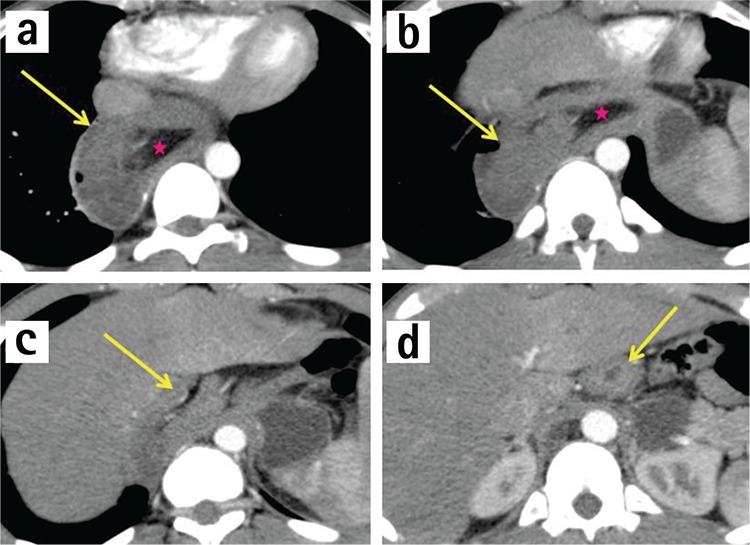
a-d. Axial view, (yellow arrows show stomach layers, red stars omental fat). Contrast-enhanced computed tomography (arterial phase) showed all layers of the stomach and omental fat herniated into the lower oesophagus through a hiatal hernia.
